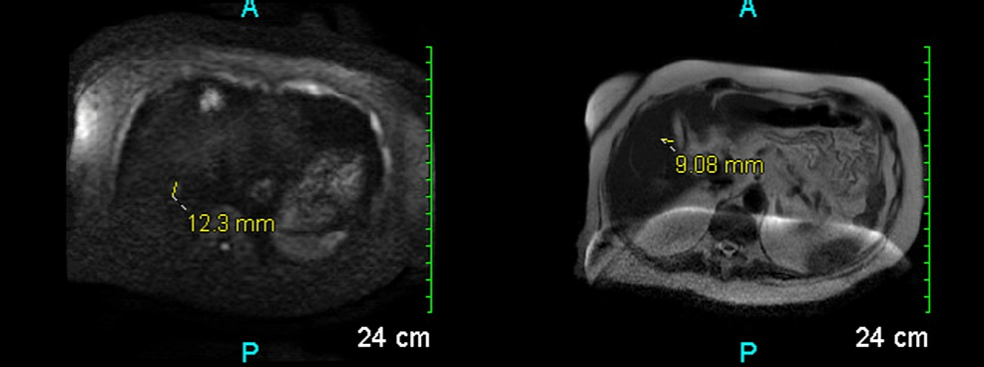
Magnetic resonance imaging revealed two echogenic foci in the liver.
